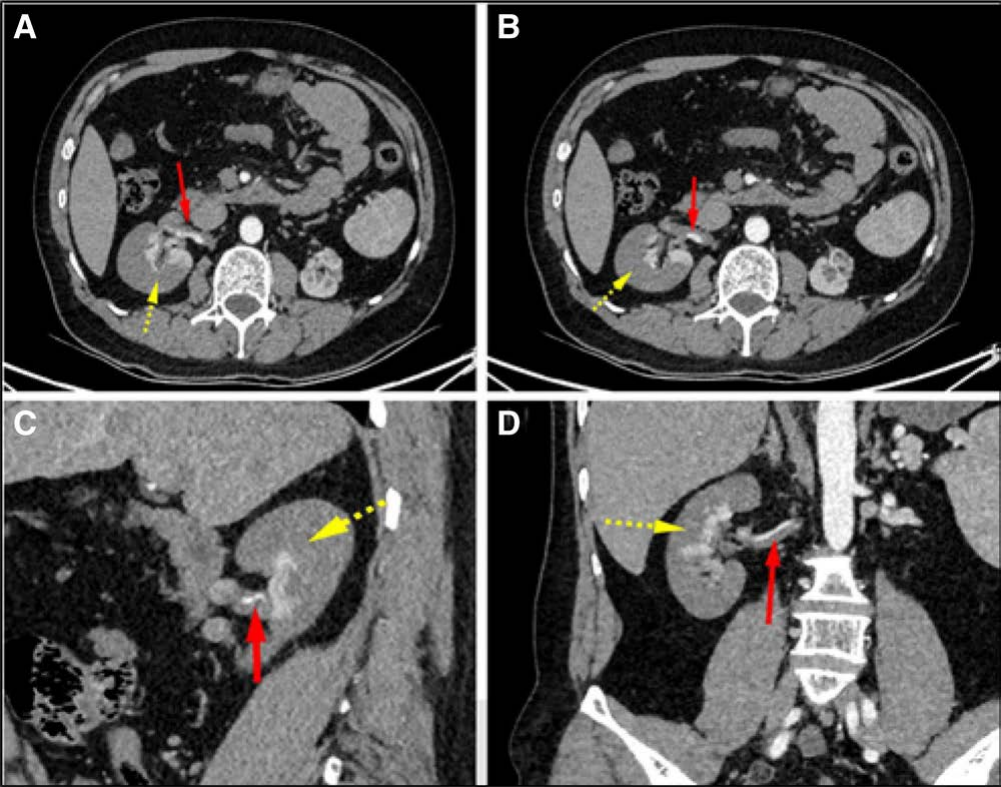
Contrast-enhanced CT scan with multiplanar reconstruction (arterial phase) revealed a linear intimal flap spanning 3.5 cm from the origin to the mid-segment of the right renal artery (Fig. 1A and B, red solid arrows), accompanied by complete absence of distal branch opacification (Fig. 1C, yellow arrows). Approximately 80% of the right renal parenchyma demonstrated wedge-shaped non-enhancement, consistent with acute total infarction (Fig. 1D, yellow dashed arrows).
